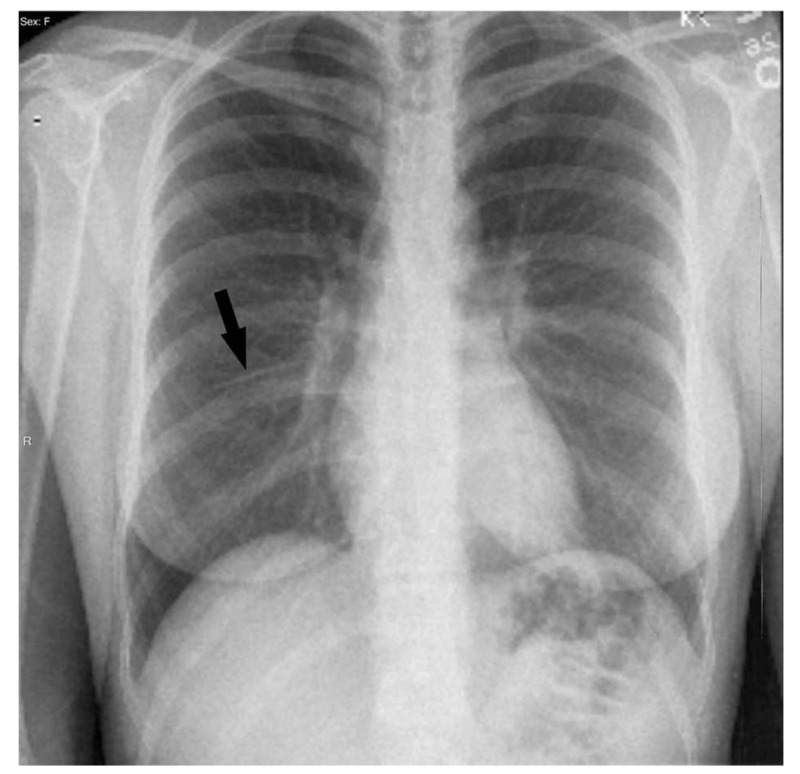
Chest X-ray showing the Nexplanon (arrow) projecting over the right perihilar lung.
